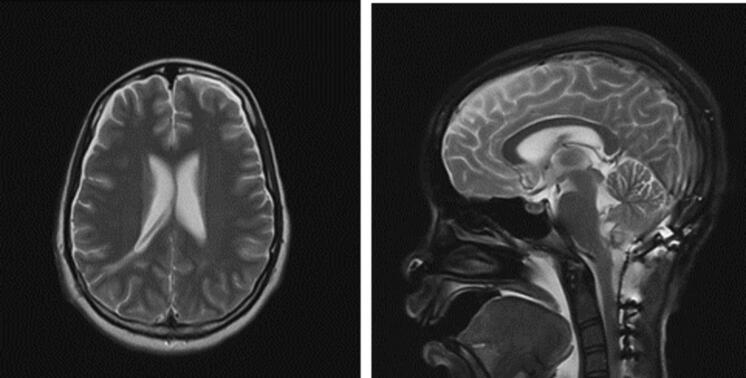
MRI brain T2 weighted (axial and sagittal views) demonstrating resolution of cerebral edema along bilateral cerebral hemispheres.
